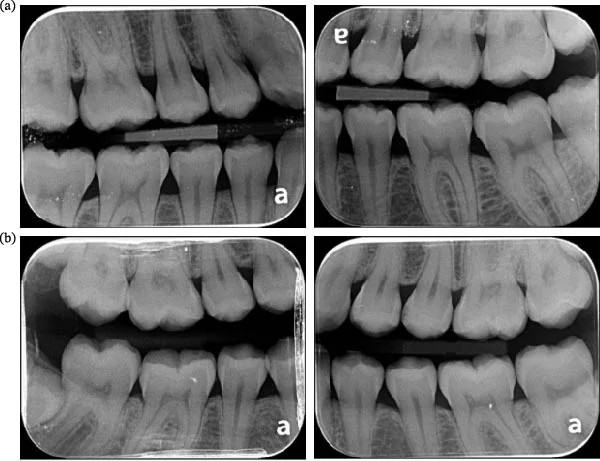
Radiographic appearance of pulp stones. Representative bitewing radiographs from study participants demonstrating radiopaque pulp calcifications (pulp stones) within the pulp chambers. (a) Bilateral first and second maxillary molars and bilateral first mandibular molars exhibiting pulp stones in a participant with documented cannabis use and clenching/grinding behaviors. (b) Bilateral first and second maxillary molars, the right shows first and second mandibular molars, and the left shows first mandibular molar exhibiting pulp stones in a participant with documented cannabis use, methylphenidate (MPH) exposure, and clenching/grinding behaviors.
